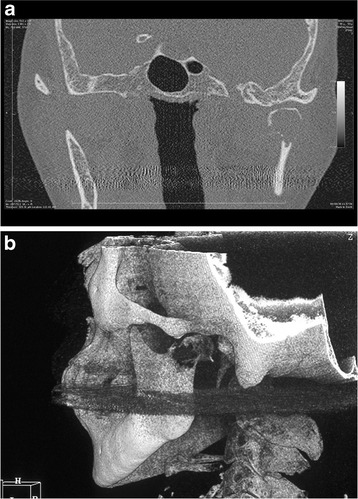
Computed tomography radiographs. a Computed tomography radiograph (coronal plane). A radiolucent destructive lesion of the left condyle was observed. The cortical bone at the condyle was thin and partially fractured. b Computed tomography radiograph (three-dimensional reconstruction). Destruction of the left mandibular condyle was observed
